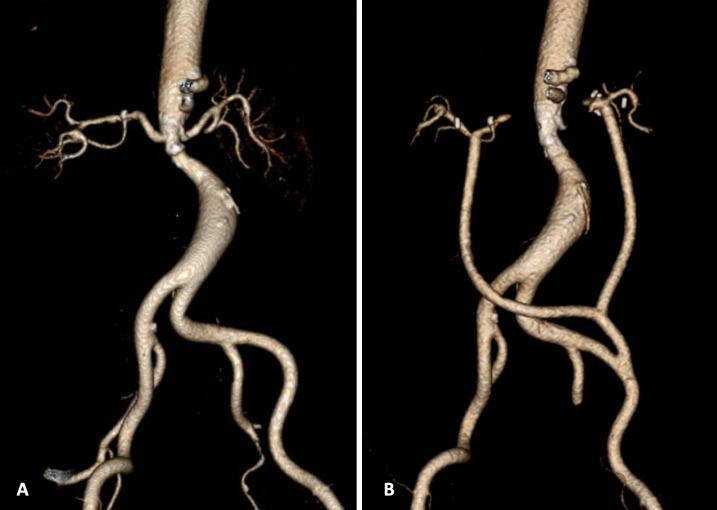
3D reconstruction of computed tomography angiography of the abdominal aorta. (A) Preoperative image showing reduction in the caliber of the aorta at the level of the renal arteries, with parietal calcifications and discrete post-stenotic dilatation; (B) Postoperative control image showing larger diameter at the level of the stenosis, with the Y-bypass to both renal arteries, from the left iliac artery.
